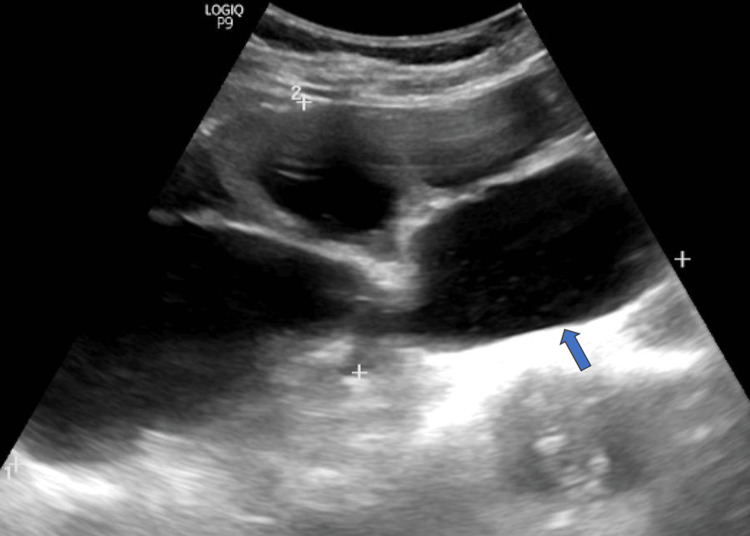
Abdominal ultrasonography showing a large cystic lesion in the pelvic region with no discernible renal cortex (blue arrow), suggestive of a grossly hydronephrotic pelvic kidney.
